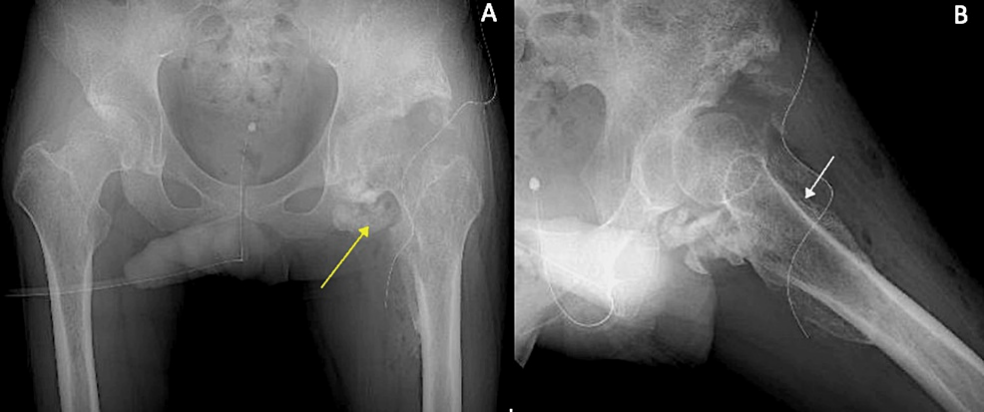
Patient 2 postoperative X-ray of the hip joint Anteroposterior (A) and lateral view (B). The yellow arrow shows the post-resection remainder of the heterotopic ossification. The white arrow depicts the extent of resection on the femoral surface in the lateral view.
